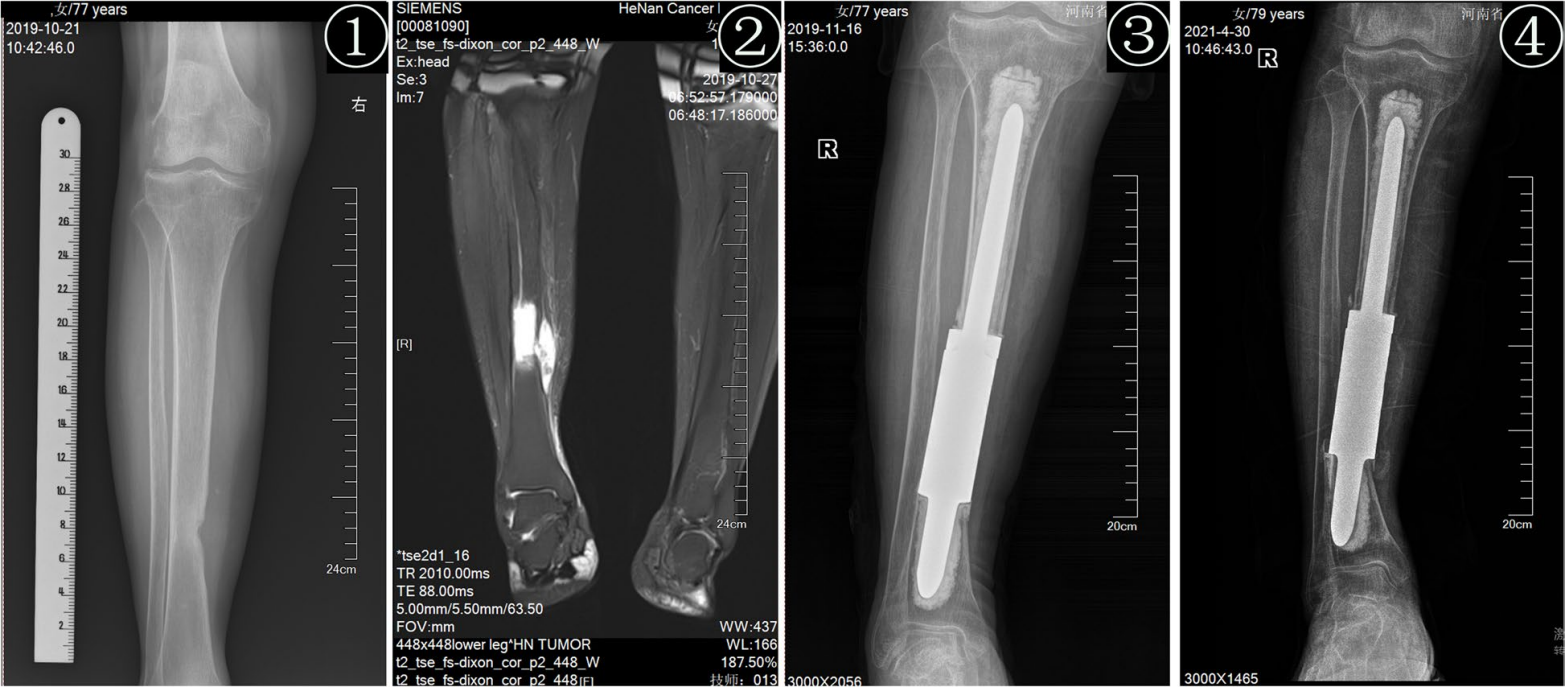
Metastatic tumor of the right distal tibia, female, 79 years old. ① the preoperative anteroposterior radiographs showed osteolytic destruction of the tibial shaft. ② Preoperative MRI T2WI (coronal) showed that the right middle tibia showed a mass-like long T2 signal, indicating the tumor signal. ③–④ The positive X-ray films of the tibial diaphysial prosthesis at 1 month and months after the operation
